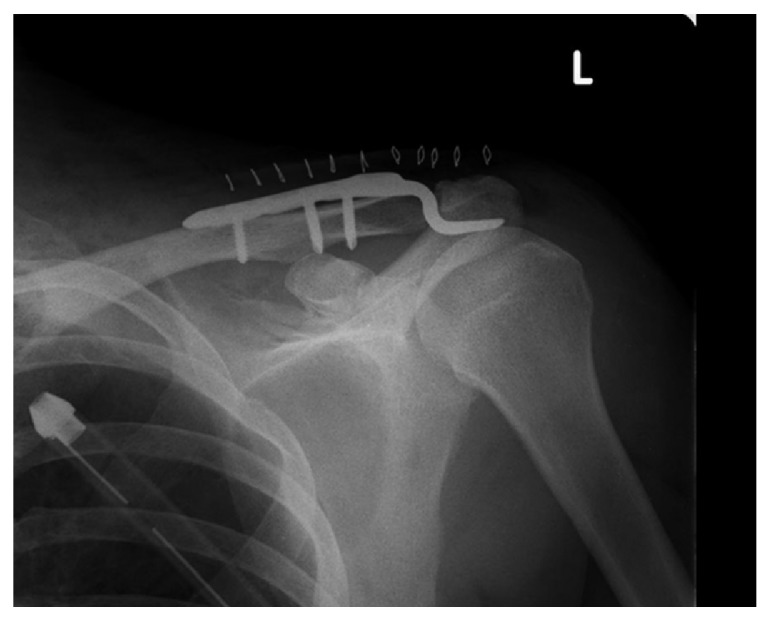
Case 2: a radiograph taken after ACJ surgery.
